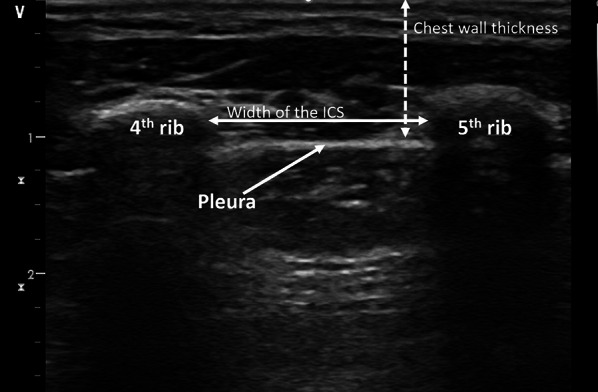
Ultrasound measurement of “chest wall thickness” and “width of the intercostal space”
